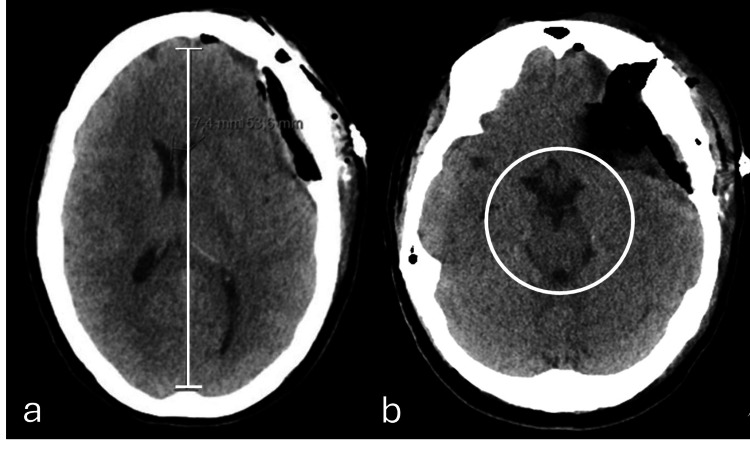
Axial plane of brain computed tomography obtained (a) post-meningioma resection (immediate post-operative phase) and (b) 24 hours post-resection without significant parenchymal changes (a) Signs of left fronto-temporal craniotomy associated with cranioplasty material (bone cement), covering a large part of the craniectomy area. It is also defined, underlying the craniotomy, as heterogeneous density material from the surgical duraplasty with small gaseous component (related to recent surgery) without significant hemorrhagic component. This material causes mass effect, with slight deformation of the adjacent parenchyma and ipsilateral ventricle, with 7 mm midline deviation of the midline structures to the right, but without significant subfalcine or uncal hernia. (b) The expansive effect exerted by these changes is unchanged, with persisting bihemispheric sulcal effacement and midline deviation of the structures to the right with attenuation of the suprasellar and perimesencephalic, which is associated with molding of the midbrain (white circle), without evident worsening compared to the prior (a) examination.
